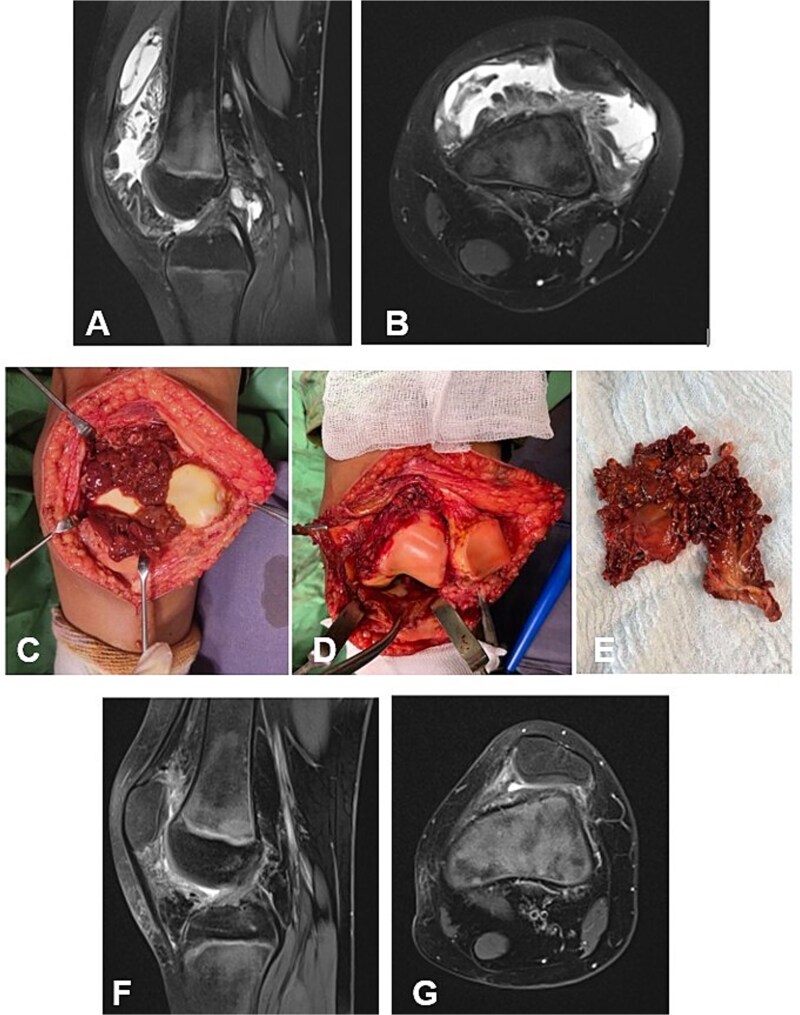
Case 2. (A and B) Preoperative Sag and Ax MRI weighted TSE PD FS. (C and E) Macroscopic appearance of the pathological synovium before and after its excision. (D) The knee joint following synovectomy. Chondral lesions correspond to Outerbridge grade I. (F and G) Postoperative 16 m. Sag and Ax MRI TSE PD FS—no evidence of recurrence of the resected neoplasm. Persistent bone marrow edema, though without clinical symptoms. PD FS, proton density weighting with fat suppression; TSE, turbo spin echo.
